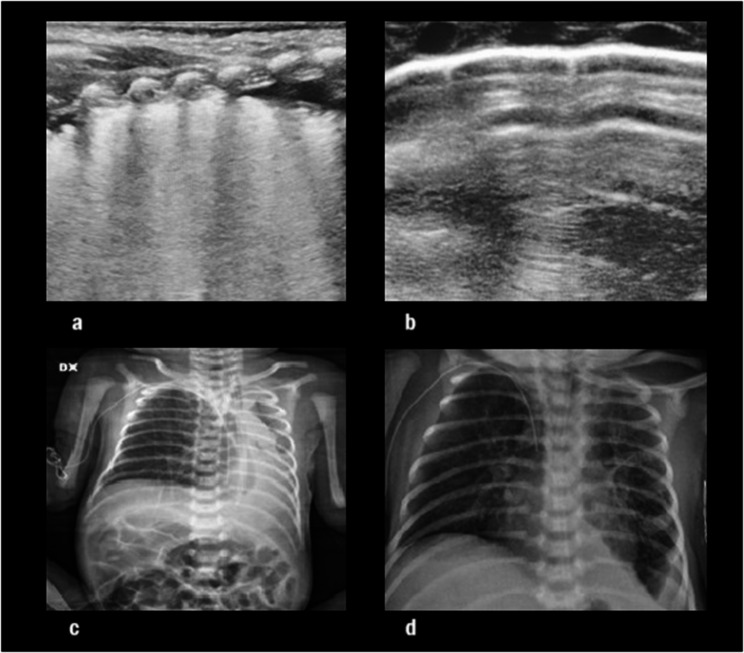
a, b, c, d. P1 LUS imaging during NIPPV (a) and sNIPPV (b); P3 chest X-ray during NIPPV (c) and sNIPPV (d)
